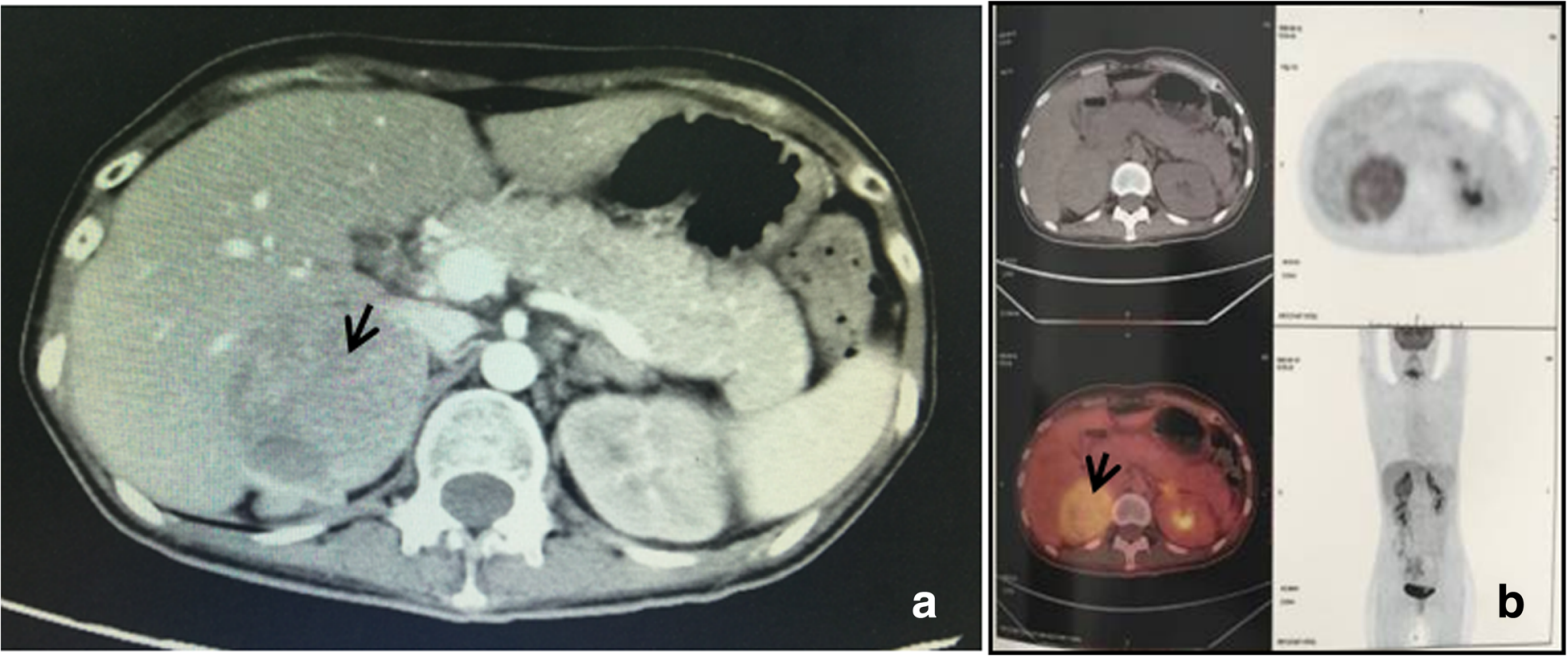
The CT and PET/CT image of the adrenal pheochromocytoma. a Abdominal contrast-enhanced CT revealing a mass with a diameter 6.5 cm in the right adrenal gland. The mass was a heterogeneous solid tumor which was mildly enhanced with some patchy nonenhancing areas (arrow). b PET/CT showed moderate FDG uptake in the right adrenal mass(arrow)
